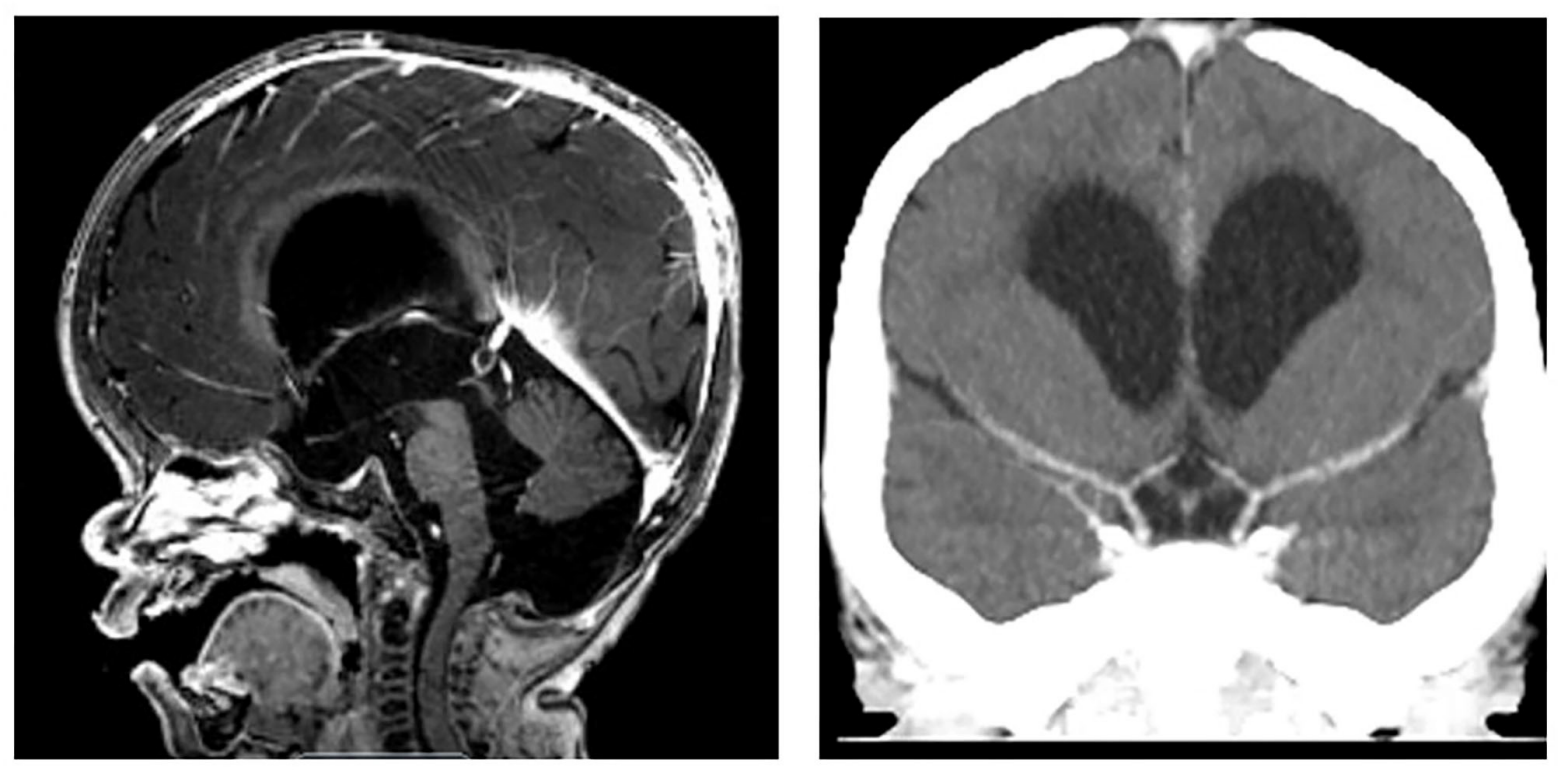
MPS I prior to stroke. (Left) MRI Head demonstrating imaging findings of macrocephaly, ventriculomegaly, small foramen magnum, and mild narrowing of cervical spine without cord compression. (Right) CT Head with contrast demonstrating bilateral internal carotid termini without stenosis.
